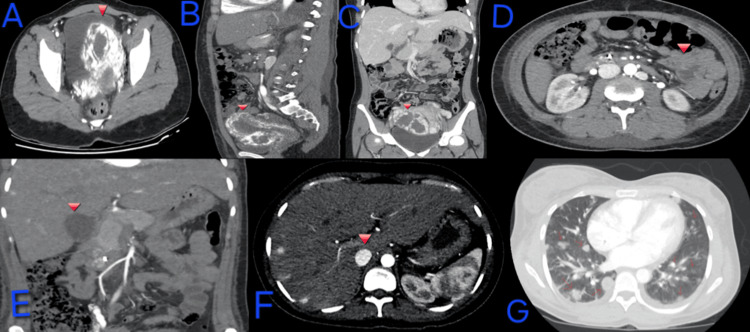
Diagnostic imaging demonstrating uterine primary lesion and multi-organ metastatic disease consistent with high-risk gestational choriocarcinoma. (A-C) Contrast-enhanced CT abdomen/pelvis demonstrating a heterogeneous uterine mass with mixed attenuation and peripheral calcifications, concerning for invasive gestational trophoblastic neoplasia.
